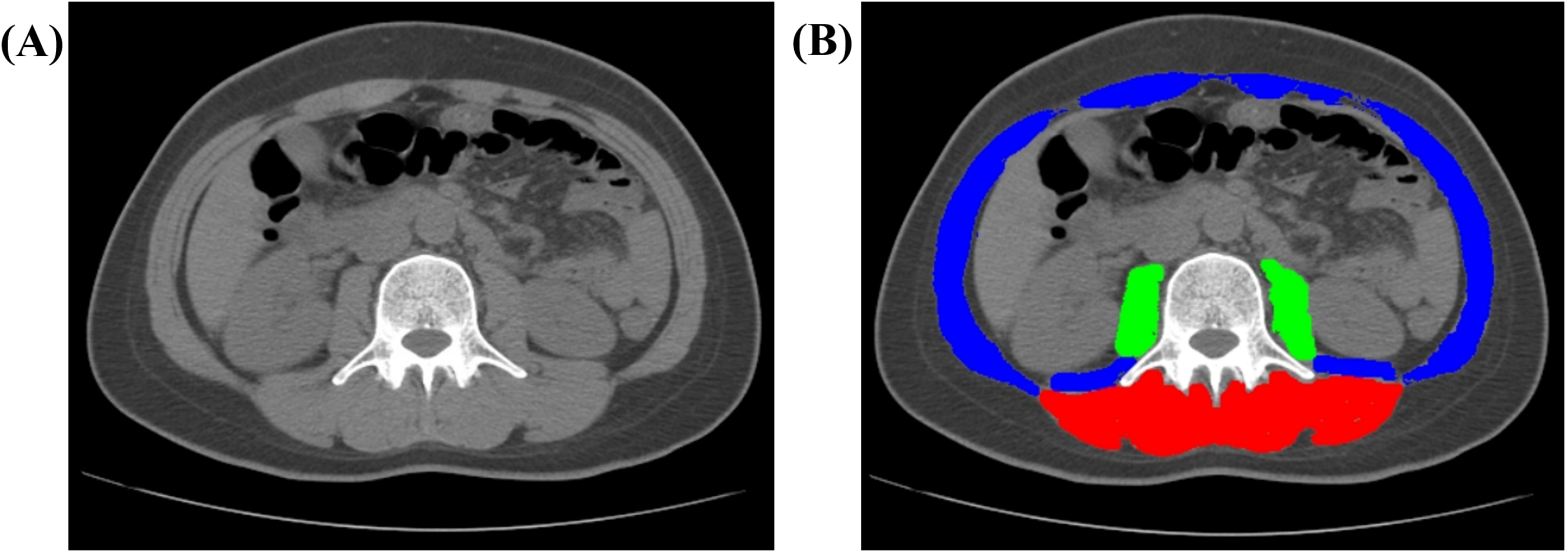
Representative CT image analysis of a 41-year-old female patient. (A) Axial non-contrast CT image acquired at the level of the third lumbar vertebra (L3). (B) Automated segmentation of muscle compartments: paraspinal muscles (PS, red), psoas major muscles (PM, green), and total abdominal muscle (TAM, combined overlay). Abbreviations: CT, computed tomography; L3, third lumbar vertebra; TAM, total abdominal muscle; PM, psoas major; PS, paraspinal muscles.
